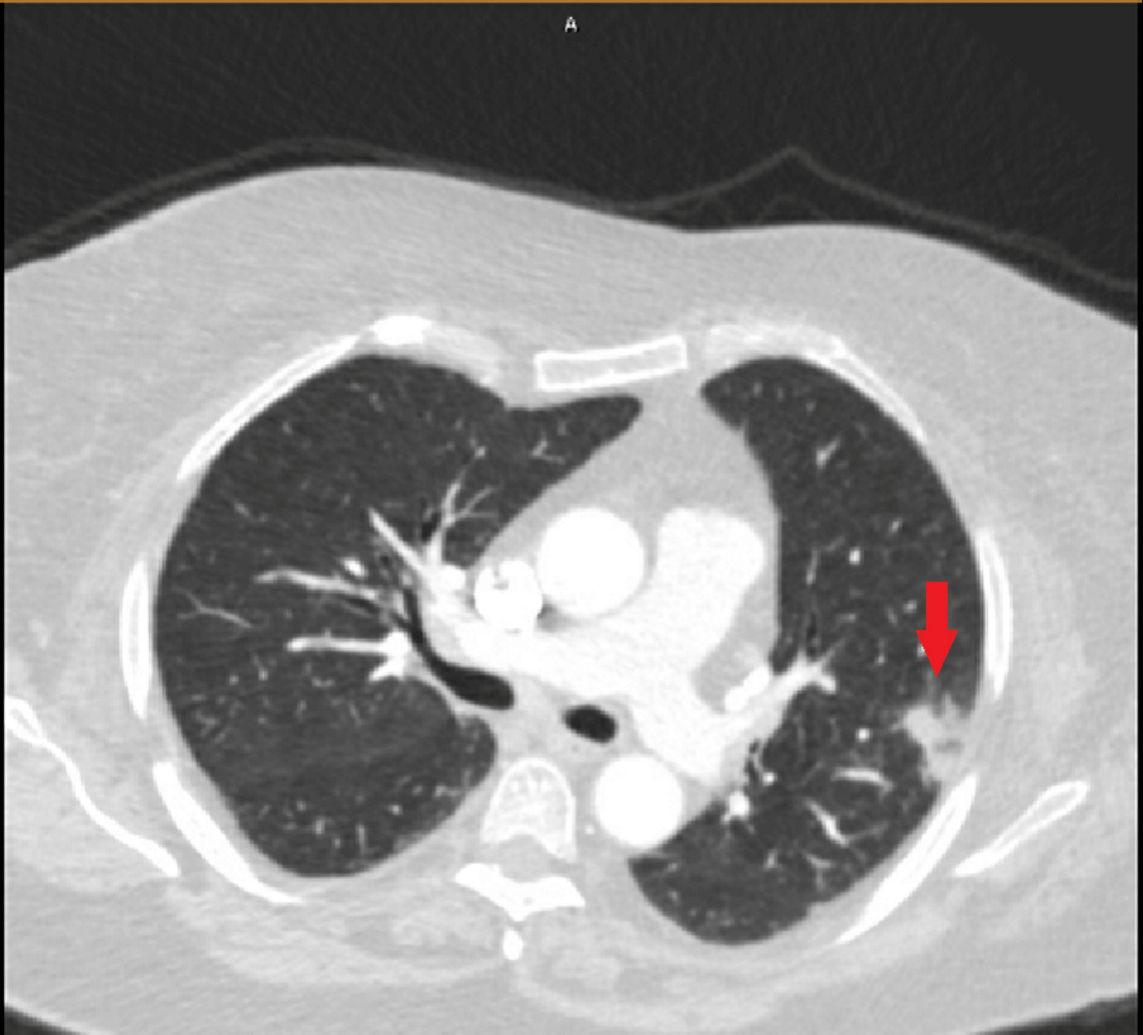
CT chest with contrast showing focal airspace consolidation in the periphery of the left upper lobe (shown by red arrow) suggestive of focal pneumonia.
